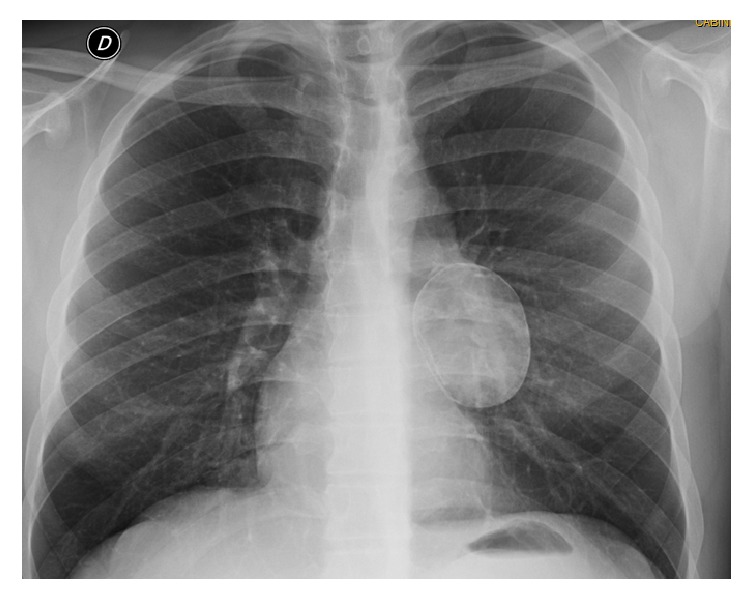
Posteroanterior chest roentgenogram elucidating a homogeneous oval opacity of water tonality, well limited, left hilar (Patient 2).
